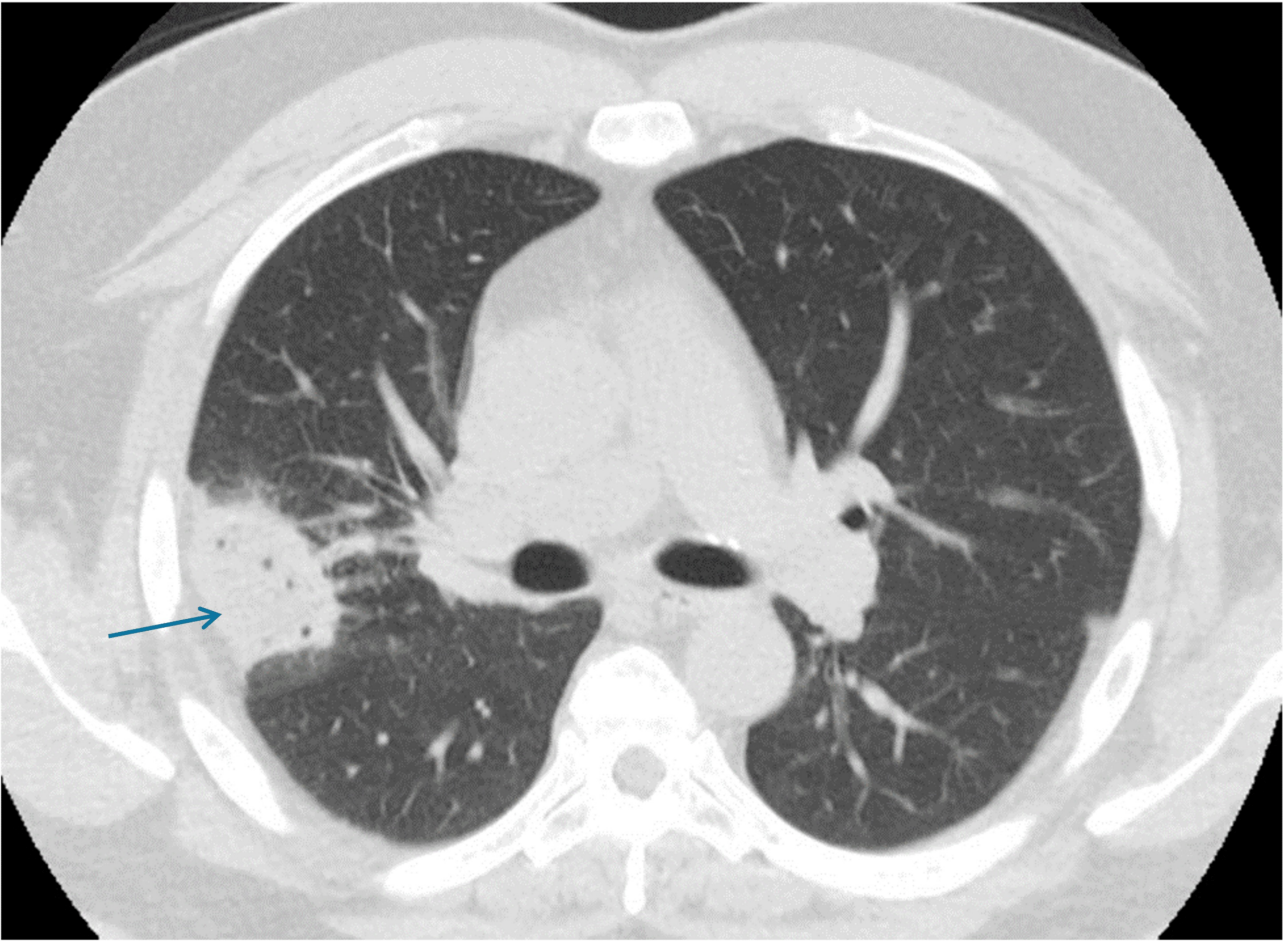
Chest CT scan image after two months Interval increased size of posterior right upper lobe (RUL) mass-like consolidation with internal lucencies, now measures 4.7 x 3.8 cm.
